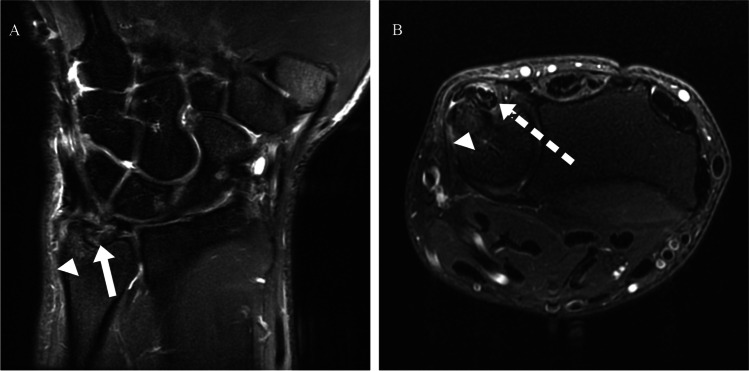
Coronal (A) and axial (B) T2-weighted fat-saturated sequences showing a peripheral TFCC tear (white arrow) with marginal ECU tendon subluxation, partial intratendinous tear and tenosynovitis (dashed white arrow), and mild ulnar styloid BME (white arrowheads)
